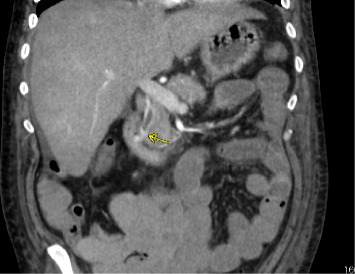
Computed tomography angiogram of the abdomen demonstrating hyperenhancement of the ampulla/papilla without active extravasation, consistent with biliary varices.
